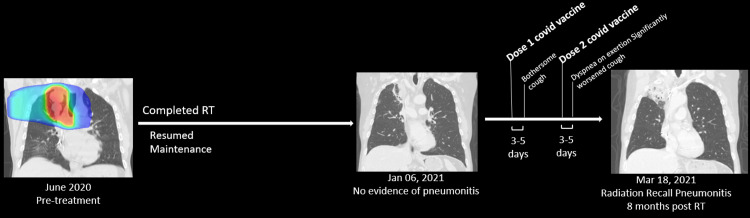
Timeline of events The patient was treated with radiation therapy and then resumed maintenance chemoimmunotherapy. Prior to the administering of the COVID-19 vaccine, there was evolving radiation fibrosis but no evidence of pneumonitis. Clinical symptoms of radiation pneumonitis began shortly after the first administration of the COVID-19 vaccine. CT imaging performed after the second dose of the COVID-19 vaccine revealed significant findings consistent with pneumonitis within the region of the previously irradiated lung COVID-19: coronavirus disease 2019
